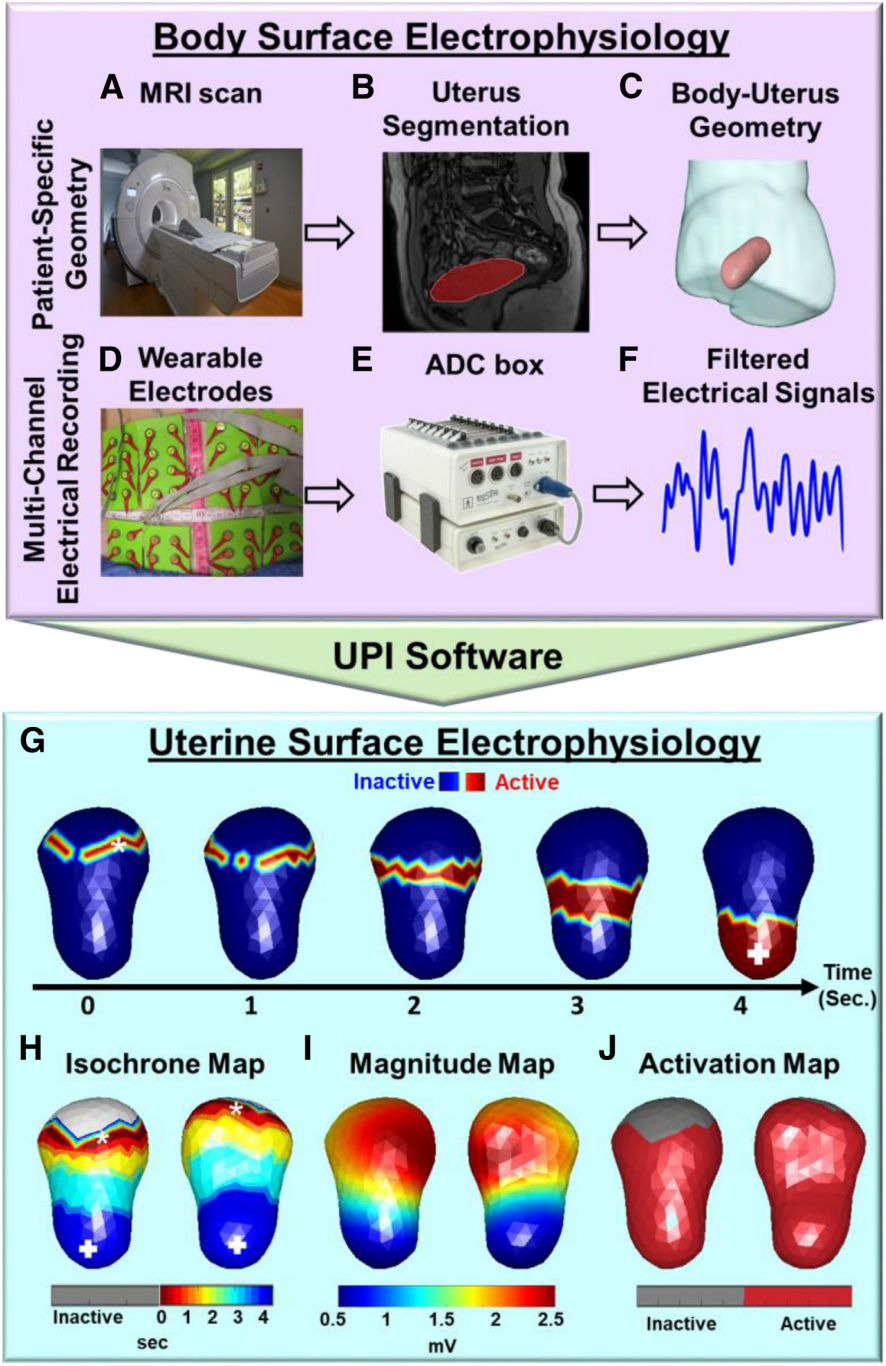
Ultrasound imaging system with automatic peristalsis detection and multiparametric quantification. (A) T1-weighted anatomical magnetic resonance imaging (MRI) scan. (B) Magnetic resonance imaging segmentation. (C) Magnetic resonance imaging–constructed body-uterus geometry. (D) Wearable electrode patches. (E) Multichannel ADC box. (F) Filtered slow-wave electrical signals (bandwidth, 0.01–0.05 Hz). (G) Electrical activation sequence of one peristalsis wave from the fundus to the cervix, with the red color representing the regions experiencing waves. (H) Isochrone map, with the gray, red, and blue colors representing inactive, early, and late activation regions, respectively. (I) Magnitude map showing the distribution of electrical potential. (J) Activation map showing the electrically activated uterine region in red. Figure 1 is adapted from the existing work (15–17), with modifications. ADC = analogue-to-digital converter; UPI = uterine peristalsis imaging.
